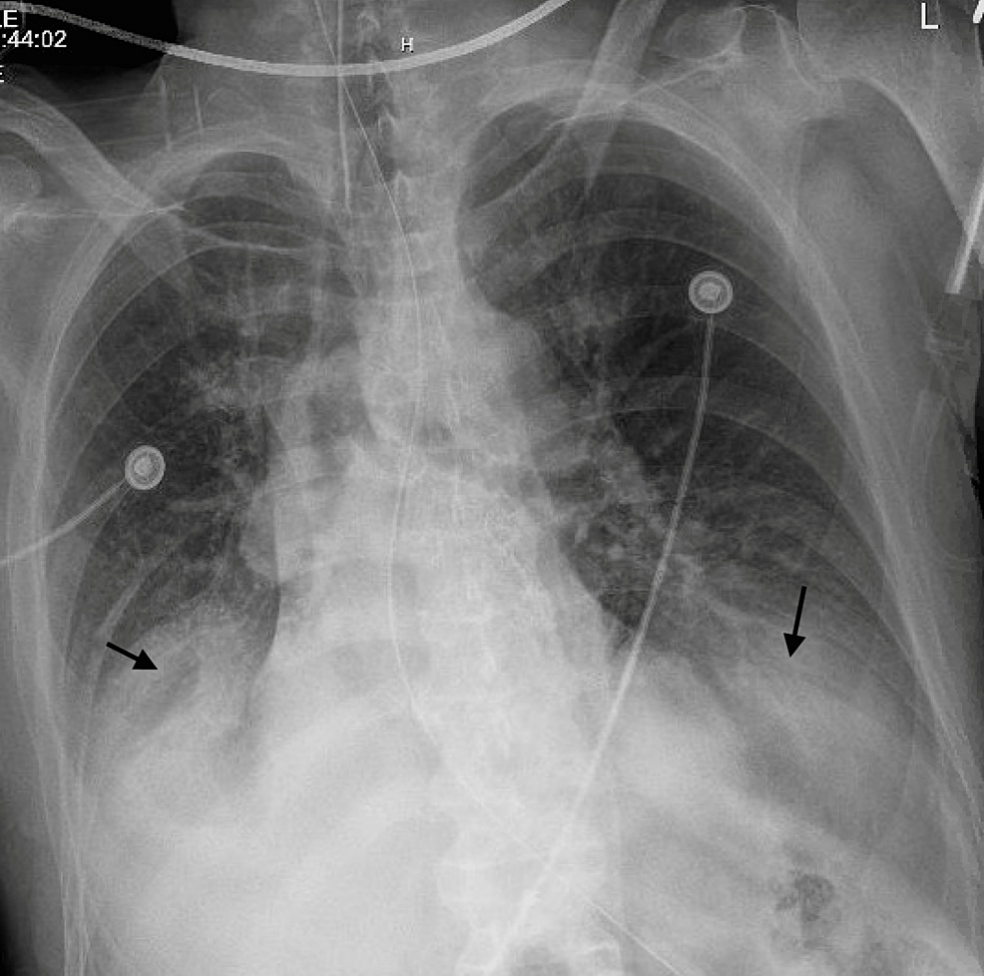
Chest X-ray after endotracheal intubation showing bibasilar atelectasis from airway obstruction.
